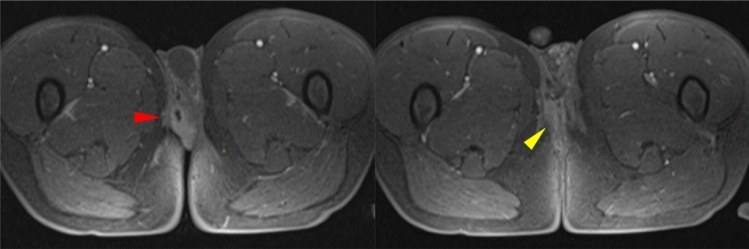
Axial MRI showing right scrotal abscess (red arrow) with a perianal fistulous tract extending to the scrotum (yellow arrow). © Cleveland Clinic Foundation 2026
